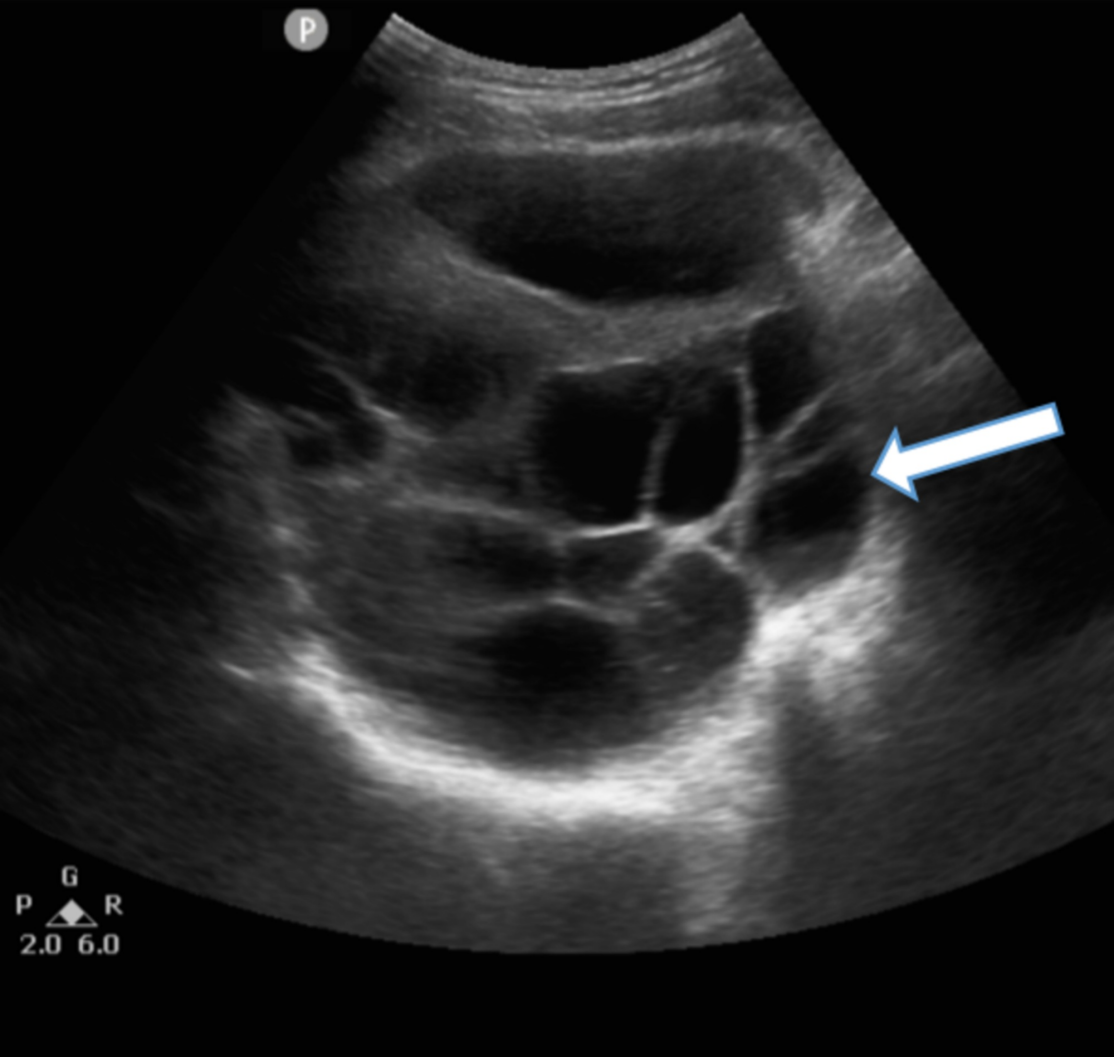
Transvaginal ultrasound displaying a cystic ovary. One of the bilaterally enlarged cystic ovaries (arrow) is shown on this transvaginal ultrasound of a 19-year-old gravida 2 para 1 female.
